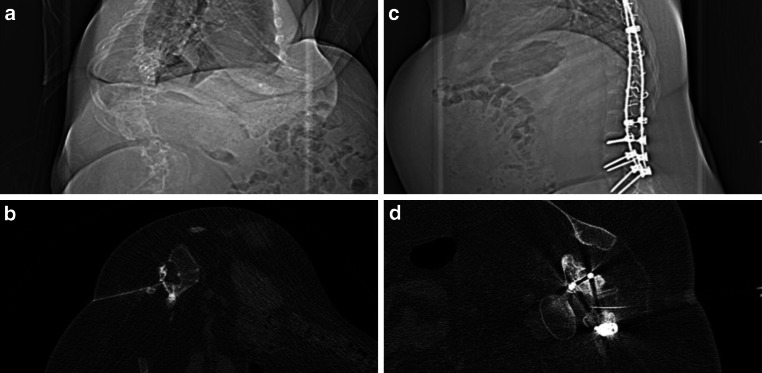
Representative CT planning images in patients with and without dorsal spinal fusion. Scout image (a) and corresponding axial CT image (b) in a patient without spinal instrumentation, showing unobstructed anatomical landmarks and a straightforward intrathecal needle path. Scout image (c) and axial CT image (d) in a patient with dorsal spondylodesis, showing increased procedural complexity due to altered bony anatomy and spinal hardware, necessitating advanced planning and angulated needle trajectory
